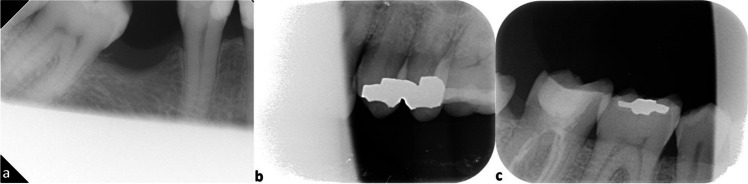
Superior premolars and molars and inferior premolars and molars radiographs with cone cutting that affects the diagnostic quality (score 2). The apex was not visible due to cone cut (a). The entire crown was missing due to cone cut (b). The bone level next to the teeth was not visible due to cone cut (c)
